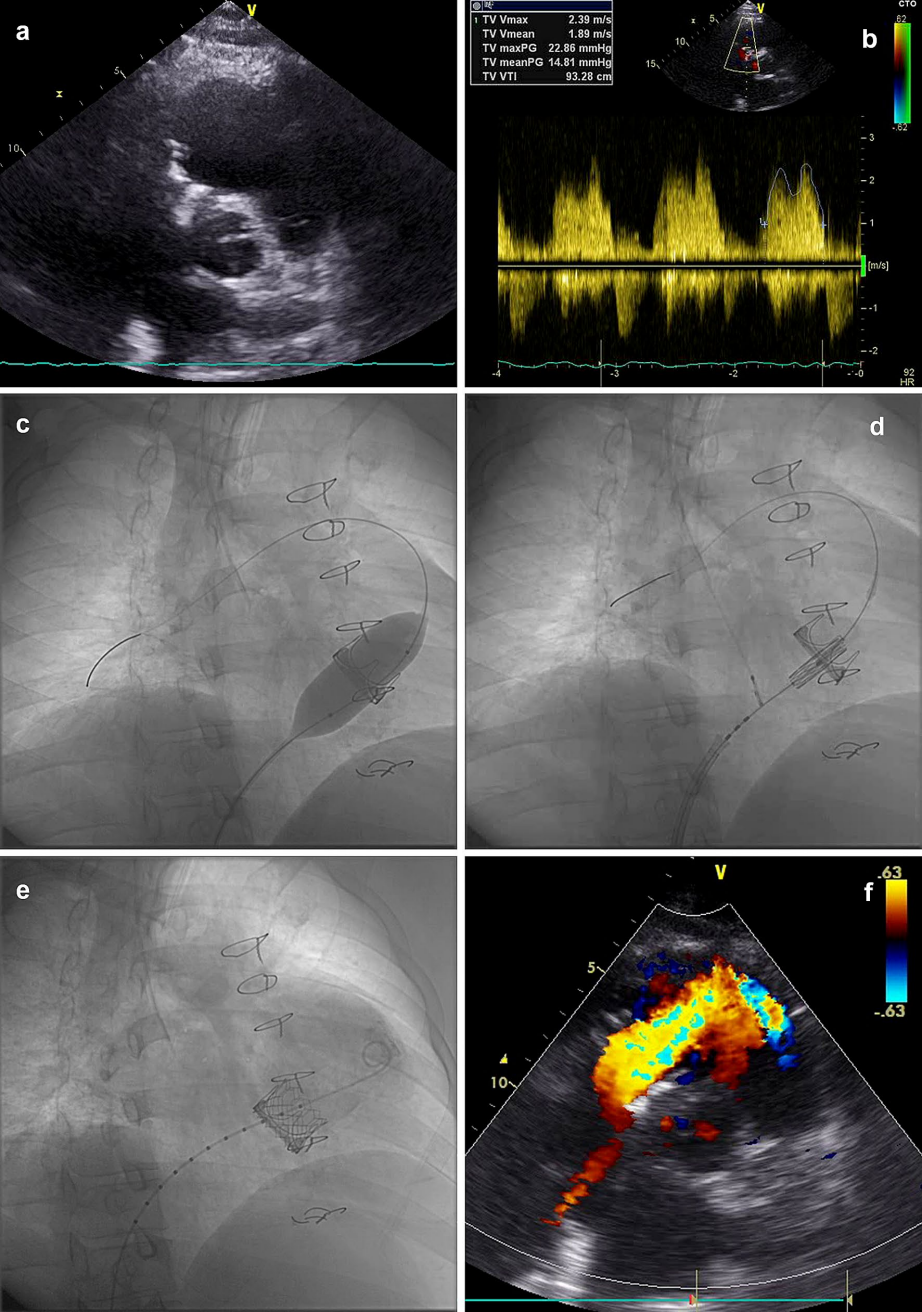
TTE, Doppler, valve placement and final colour Doppler
